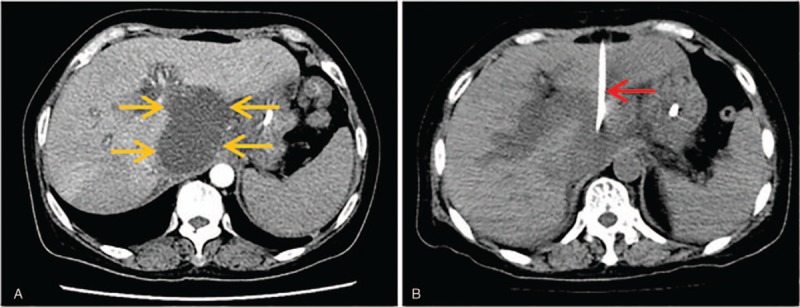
Transhepatic third segment approach. (A) Axial CT scans showed an abscess (light orange arrow) with size of 6 × 8 cm was detected in the lesser peritoneal sac. (B) Axial CT scans showed drainage catheter (red arrow) get through the third segment of liver parenchyma into the abscess cavity and abscess cavity completely collapsed ten days after the placement of drainage catheter.
